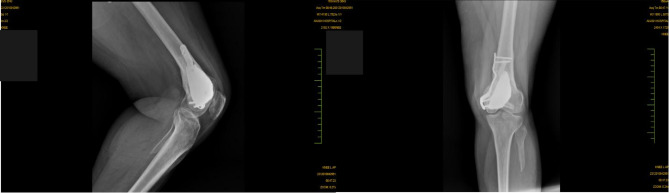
Radiographs taken at 6 months post-surgery. It shows complete structural integration of the affected area. The images confirm that the healing process is fully completed with no recurrence of complications. This stage marks the successful restoration of the patient’s mobility
